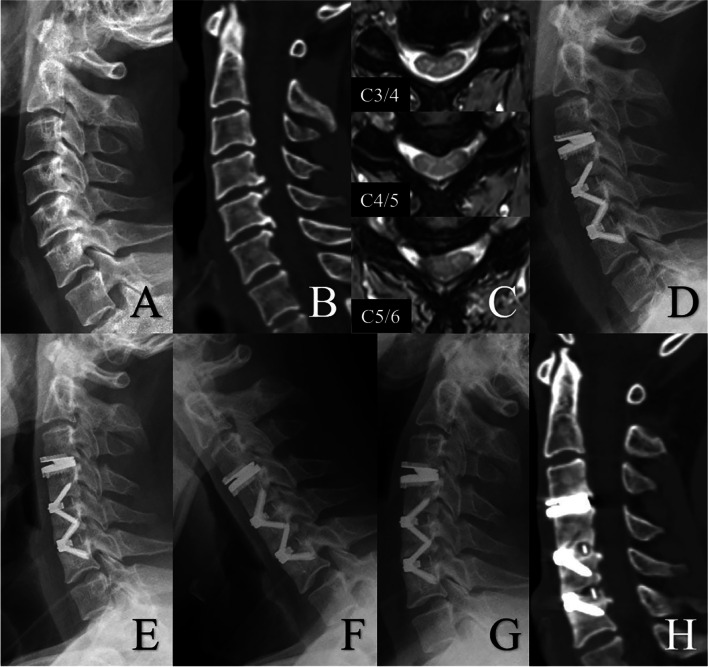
Radiologic examinations of a 45-year older woman with neck pain for 1 year, who had cigarette consumption for more than 10 years. Preoperative lateral X-ray showed good cervical lordosis (A). However, a sagittal CT scan showed osteophytes at the posterior border of C4/5 and C5/6 (B). MRI demonstrated spinal cord compression at C3/4, C4/5 and C5/6 (C). The patient underwent HS, including CDR at C3/4, and ACDF at C4/5 and C5/6 (D). At 1-year follow-up, lateral X-ray shows satisfactory cervical lordosis (E), and extension-flexion view showed good cervical ROM (F and G). However, a postoperative CT scan showed incomplete bony fusion at both two arthrodesis levels (H)
